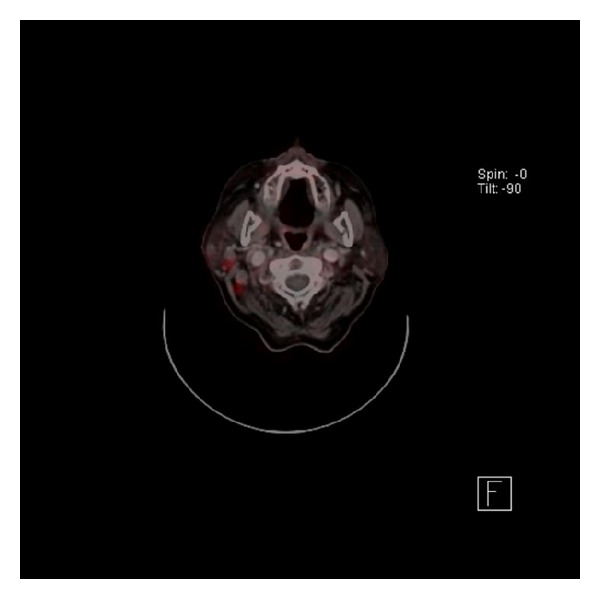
PET/CT: pathologic enhancement in the right parotideal region.
